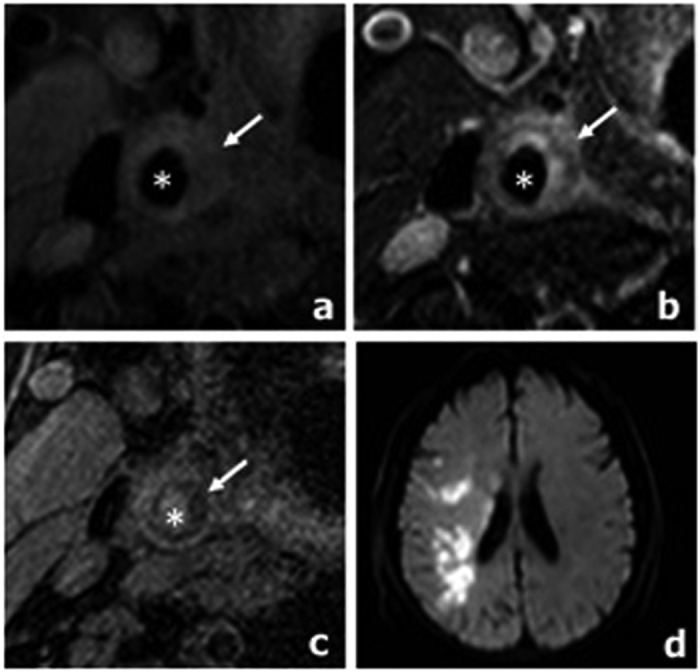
Representative MR images of a subject with high level HbA1c (HbA1c = 7.8%). (a–c) An atherosclerotic plaque with large LRNC (arrow) is detected in the right carotid artery (star): iso-intensity on T1-weighted image (a); hypointensity on corresponding T2-weighted image (b); hypo-intensity on MP-RAGE image (c). (d) Cerebral DWI demonstrates ACIs (hyperintensity) at right hemisphere.
